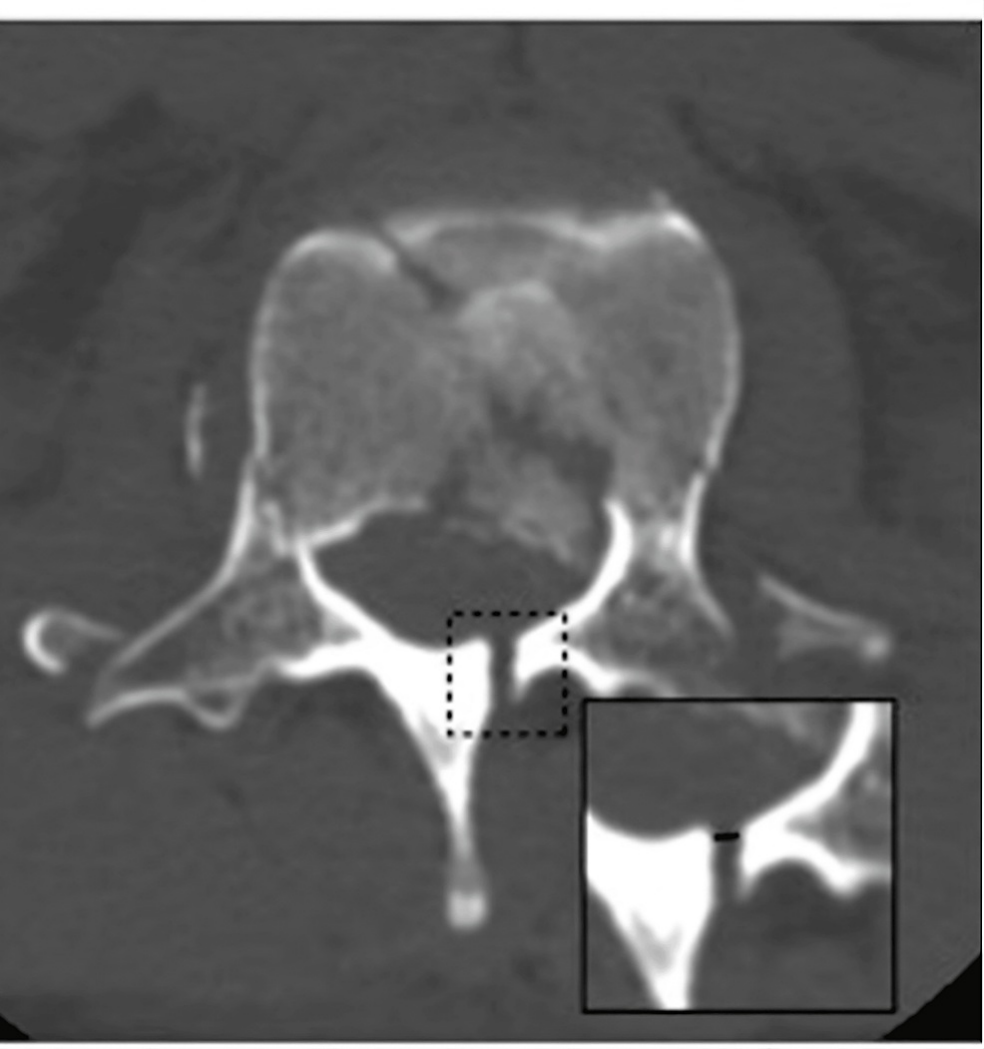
The presence of laminar and pedicle fracture is identified and the distance of the separated lamina is measured in CT axial plane.
